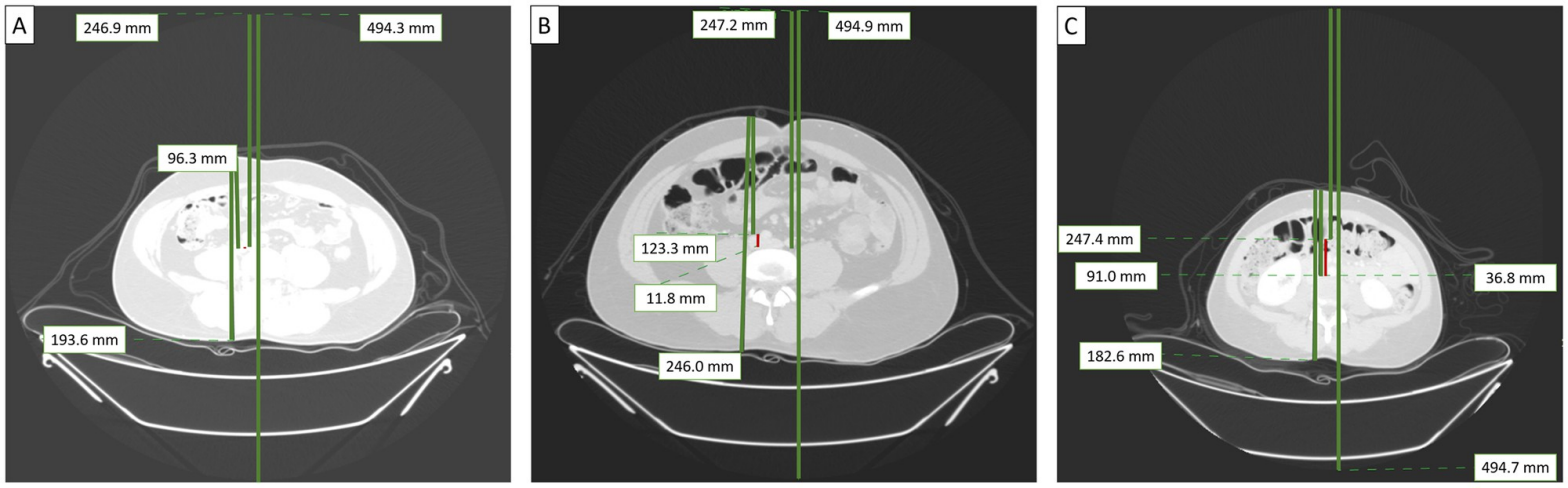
Transverse CT images of three patients reconstructed with full field of view (FOV). Patient A was centered optimally in the CT gantry. Patient B was centered above the gantry isocenter (+11.8 mm) while patient C was centered below the gantry isocenter (–36.8 mm).
